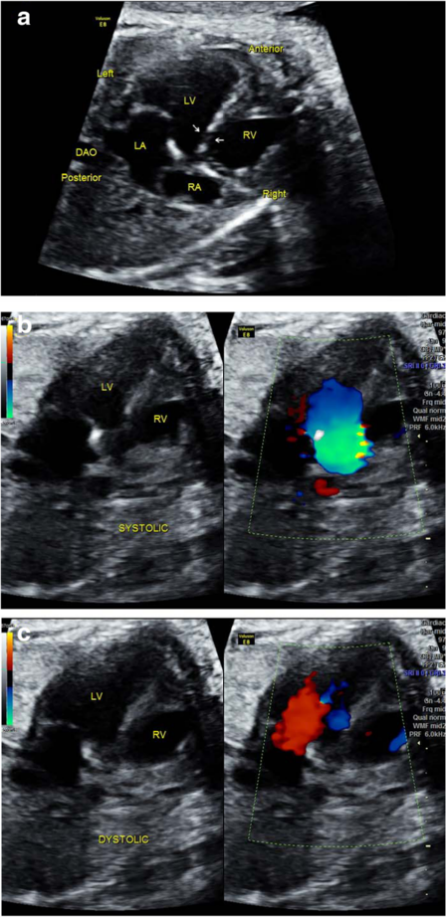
Sonographic images of a 24-gestational-week fetus in which VSD was falsely suspected. In the 4CV, a small echo drop-out was visualized at the perimembraneous septum, as indicated by the white arrows (a). This was at times easily considered to be a VSD, whereas it was actually an artifact. Color Doppler helped to resolve this problem. No flow signals were identified across the septum in either systolic (b) or diastolic (c) phases
